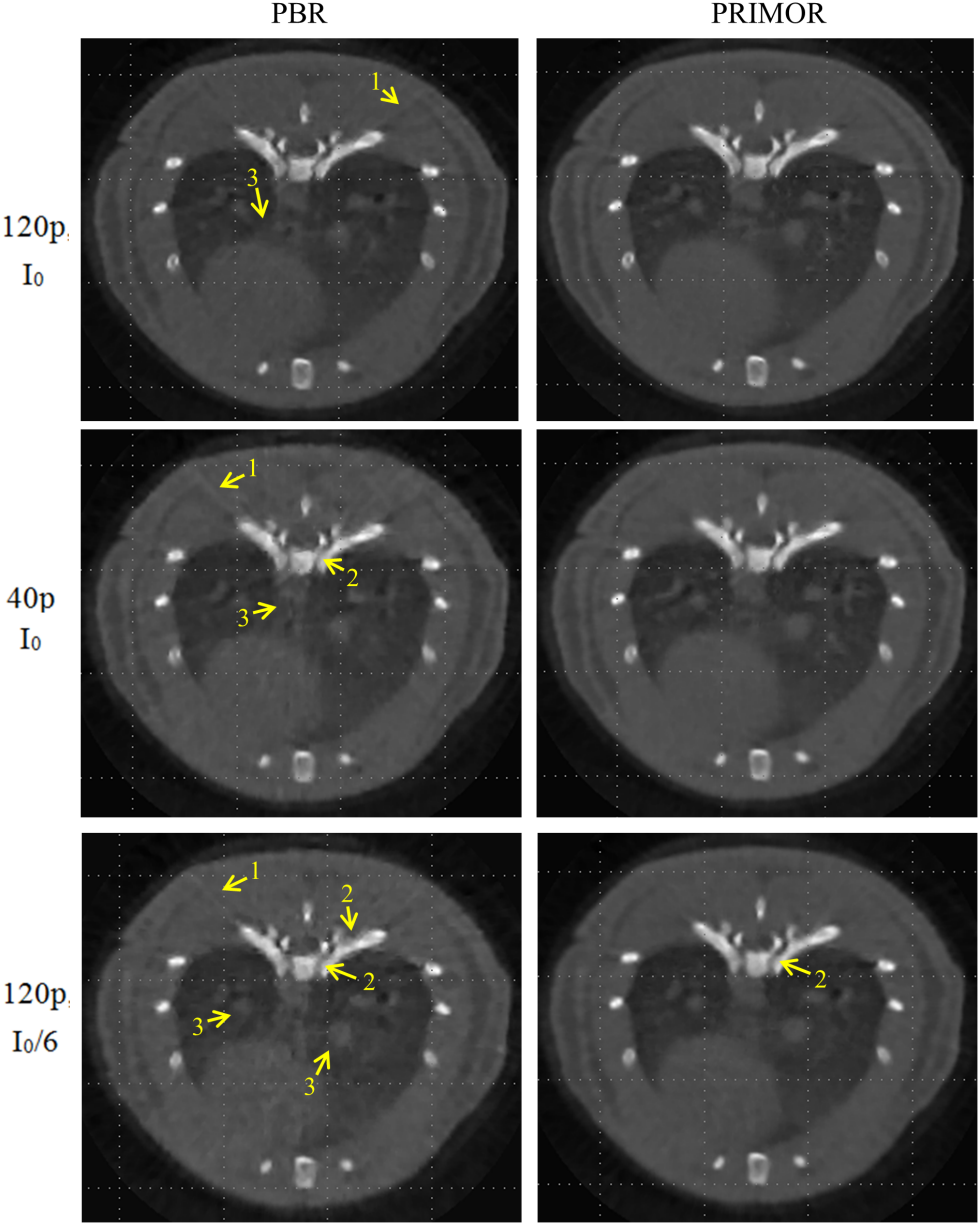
Images corresponding to different scenarios for respiratory phase three, reconstructed with PBR and PRIMOR algorithms. From top to bottom, each row represents a different scenario: 120 projections and dose corresponding to a maximum number of photons I_0_ (subsampled scenario), 40 projections and I_0_ (subsampled scenario), and 120 projections and number of photons I_0_/6 (low-dose scenario). Yellow arrows indicate where artifacts are more noticeable: an increase in streak artifacts (1), blurred edges for bone tissue (2), and blurred edges for soft tissue (3). Videos for these results showing images for all gates are available at https://github.com/HGGM-LIM/prior-motion-reconstruction-CT.
